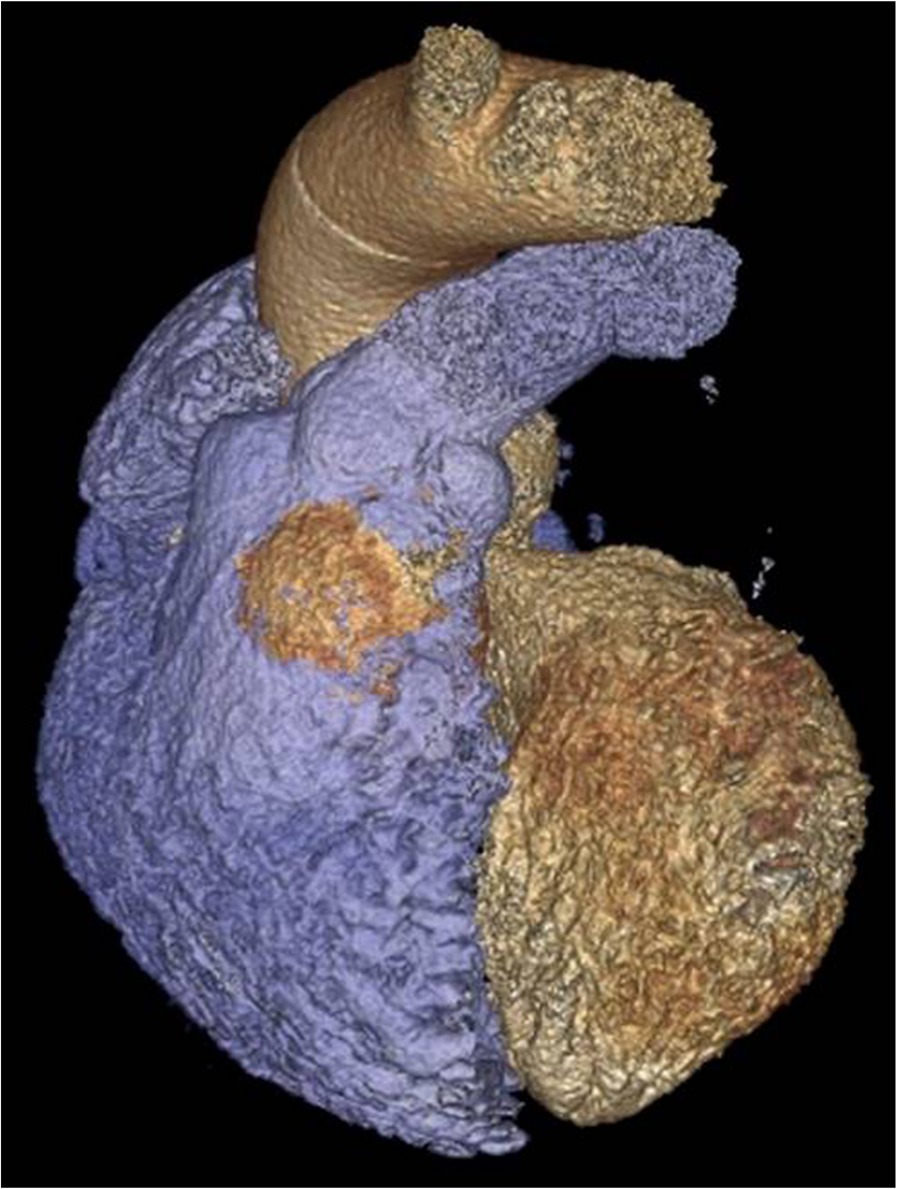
Volume rendering of the CTA scan indicating the SVA in brown colour within the right ventricle in blue
